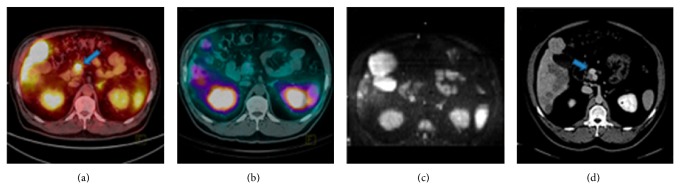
A 49-year-old man with NET of unknown origin for over 4 years. ^68^Ga-DOTATATE PET/CT (a) identified primary pancreatic lesion (arrow), whereas SPECT/CT (b) and WB DWI (c) did not. This lesion was noted only retrospectively (arrow) on dedicated abdominal CT (d) performed 4 years previously [13].
